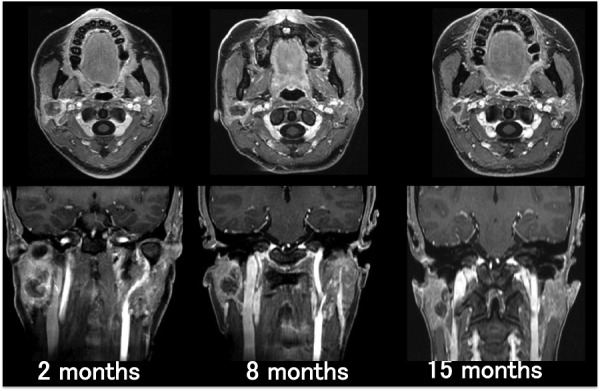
Time course of axial (upper row) and coronal (lower row) T1-weighted with Gd-enhancement. Over the 15-month follow-up period, the mass in the right parotid shows a decrease in size.
